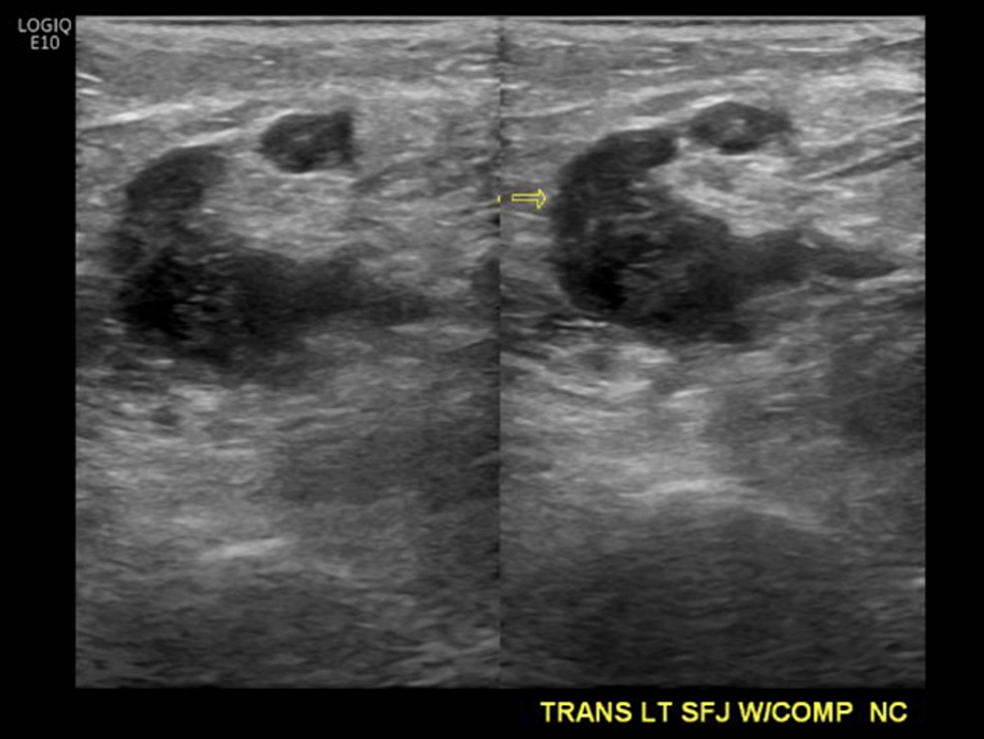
Lower extremity venous Doppler. Left non-occlusive DVT in saphenofemoral junction. DVT, deep venous thrombosis.
